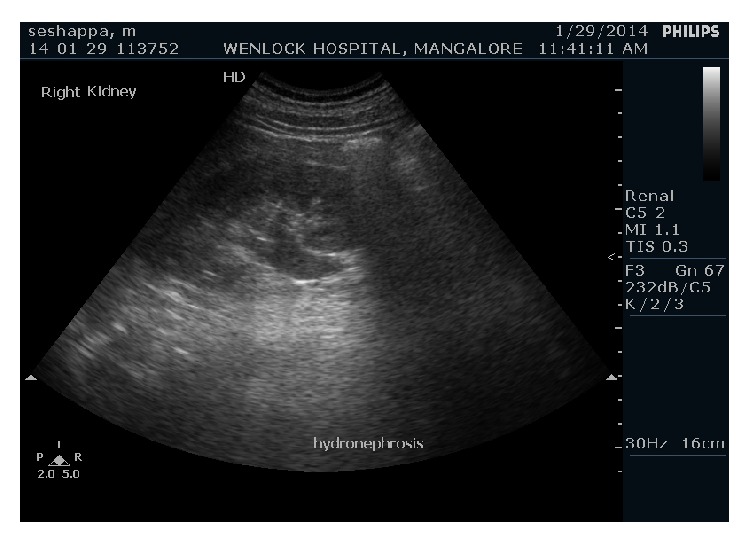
Grey scale ultrasound examination of abdomen shows dilated right renal ureter, renal pelvis, and right hydronephrosis.
